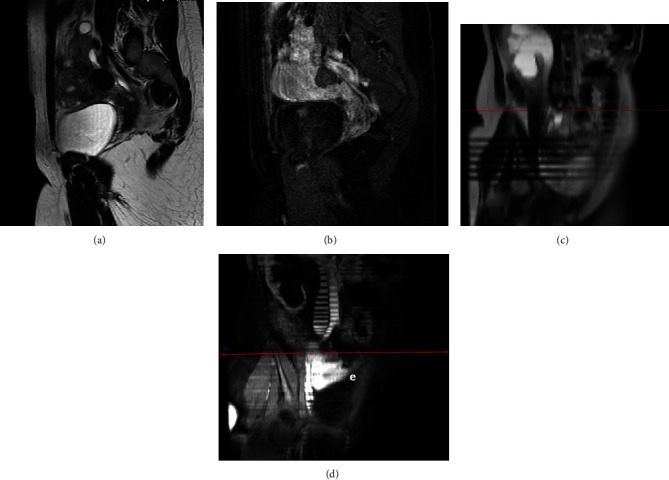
Pelvic magnetic resonance imaging showing the following: (a) sagittal T2-weighted spin-echo image: a round mass with very low homogeneous signal intensity in its central part is present; (b) sagittal 90 second postgadolinium T1-weighted fat-suppressed spin-echo image: the upper aspect of the mass enhances reflecting active disease and the nodular desmoid tumor exhibits minimal enhancement confirming its fibrous nature; (c) paracoronal T2-weighted; and (d) postgadolinium T1-weighted images showing right kidney hydronephrosis due to an extraureteral obstruction confirmed to be a desmoid tumor.
